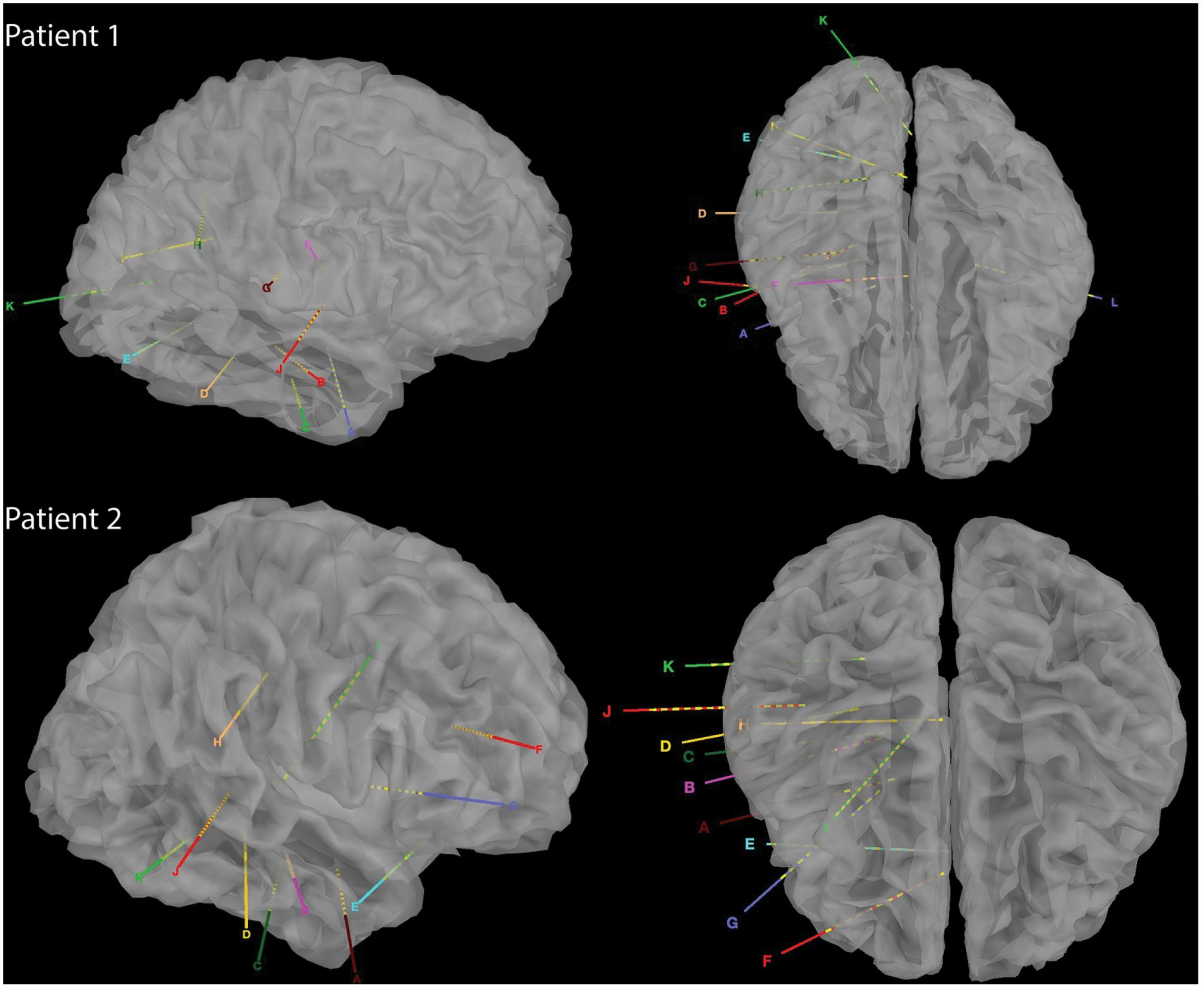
Reconstructed CT images of sEEG implants in the two patients.
